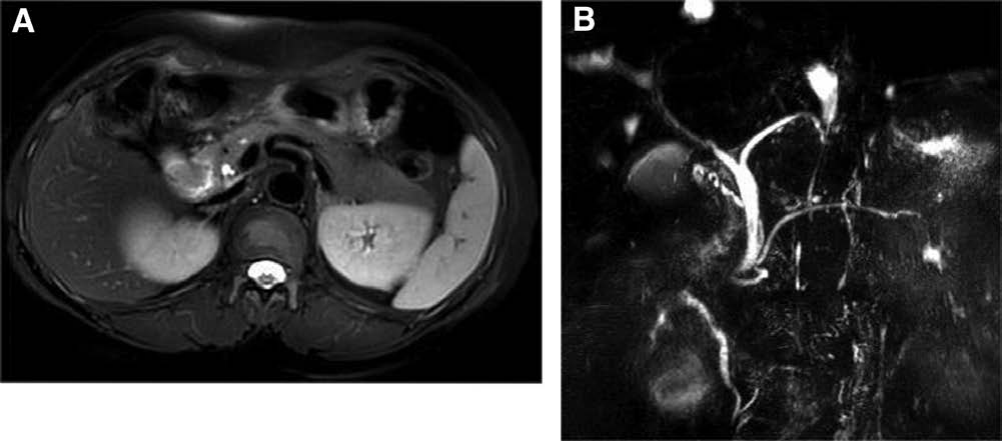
MRI and MRCP of the upper abdomen. (A) MRI enhancement. (B)MRCP. MRCP = magnetic resonance cholangiopancreatography, MRI = magnetic resonance imaging.
